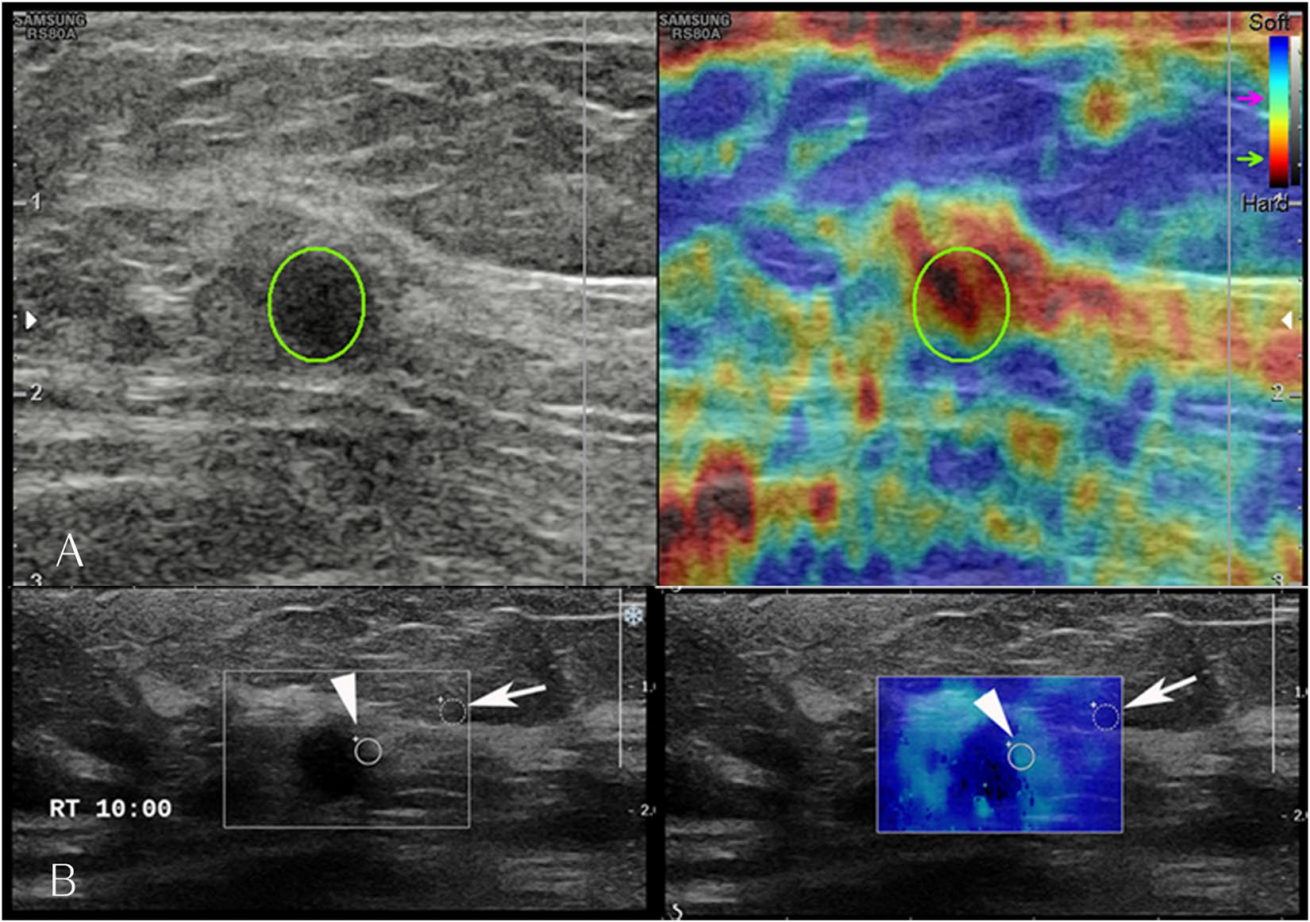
Representative images showing the region of interest used to obtain the strain ratio and elasticity ratio. (A) An oval region of interest (ROI) was set to include the mass (circle), in which the ultrasound machine automatically calculated and visualized the strain ratio as the mean strain within the ROI drawn along the border of the mass divided by the mean strain of the fat located at and above the level of the ROI set for mass strain measurement; the strain measured within the ROI set for mass measurement was excluded. (B) The elasticity ratio was calculated as the target round, solid ROI, located within the stiffest part of the lesion (arrow head) divided by another reference ROI in the adjacent subcutaneous fat (arrow).
